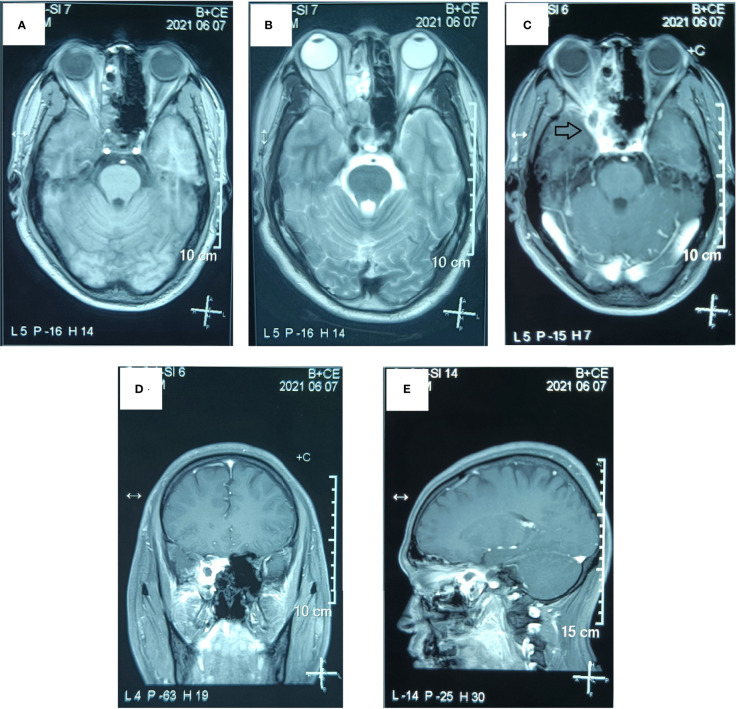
Postoperative MR examination of the paranasal sinus. The MR examination detected a residuary tumor in the surgical area and the lateral side and the tip of the right orbit. (A) Axial T1-weighted image. (B) Axial T2-weighted image. (C) Axial T1-weighted enhanced image. (D) Sagittal T1-weighted enhanced image. (E) Coronal T1-weighted enhanced image. (The mass is marked with a black arrow.).
